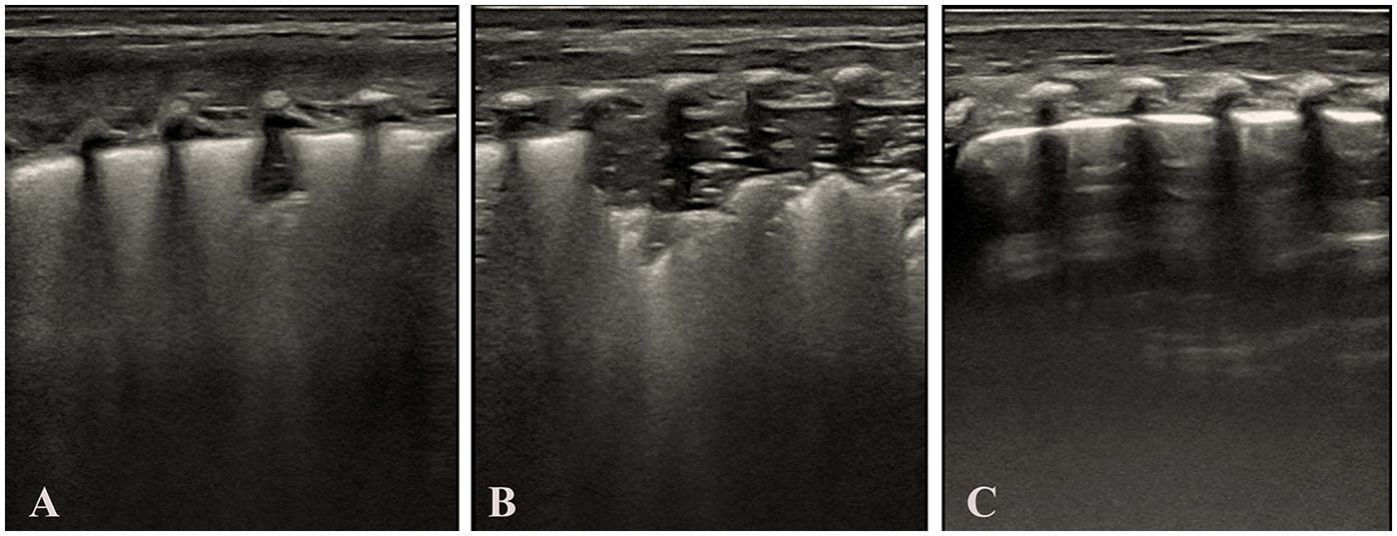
Demonstrates LUS imaging and PS treatment response of TTN infant with pulmonary consolidation. (Panel A) Initial LUS demonstrates features consistent with the alveolar interstitial syndrome (AIS), including a blurred pleural line and absence of consolidation. (Panel B) Subsequent LUS shows the development of pulmonary consolidation, bronchial inflation sign, absent A-lines, and an irregular pleural line. (Panel C) LUS following surfactant administration, showing resolution of consolidation, a normal pleural line, and the reappearance of A-lines. Images were acquired using a Mindray M9 portable color Doppler ultrasound system (Shenzhen Mindray Biomedical Electronics Co., Ltd., China).
